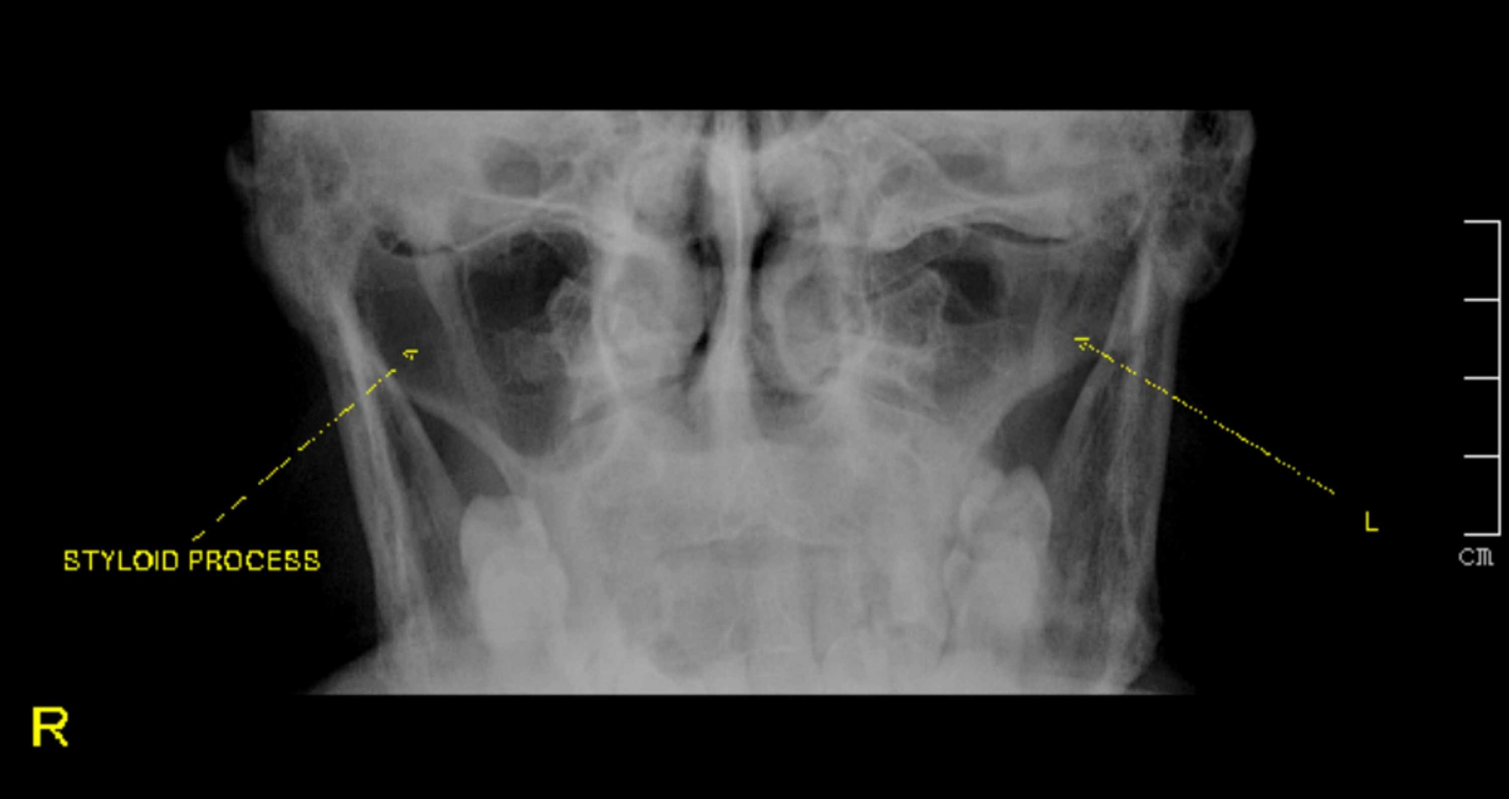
X-ray of the skull (Towne's view) showing an elongated styloid process on the right side and a normal styloid process on the left side
